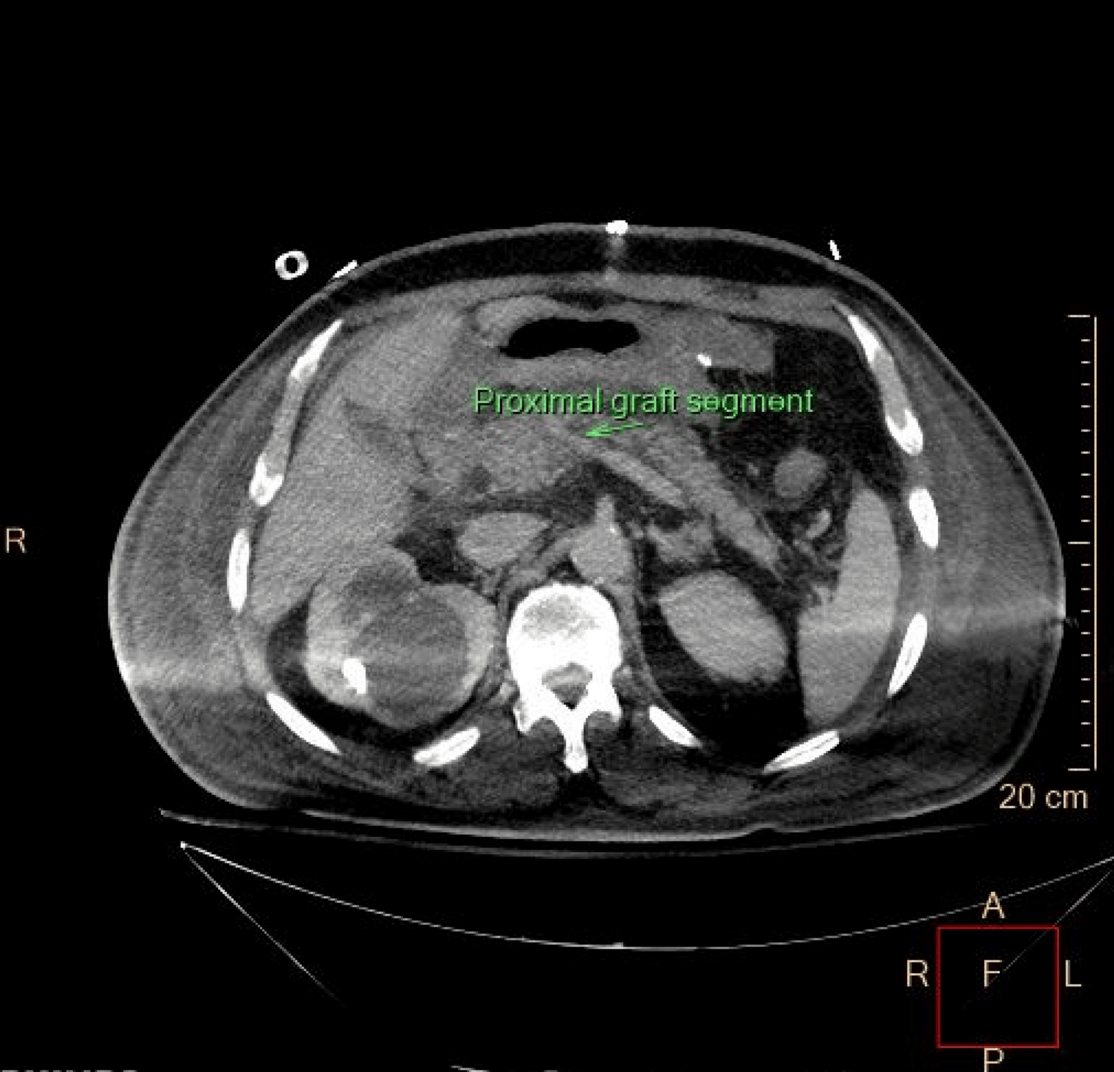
Postoperative CT scan confirming the patency of the graft The arrow shows the proximal end of the graft.
